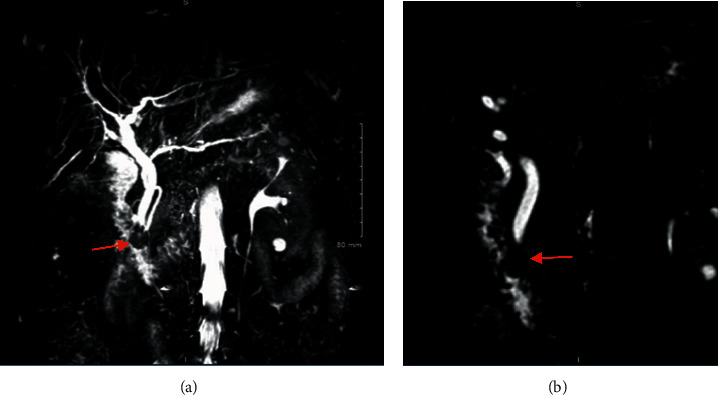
MRI/MRCP showing CBD dilatation without choledocholithiasis as well as papillary stenosis.
